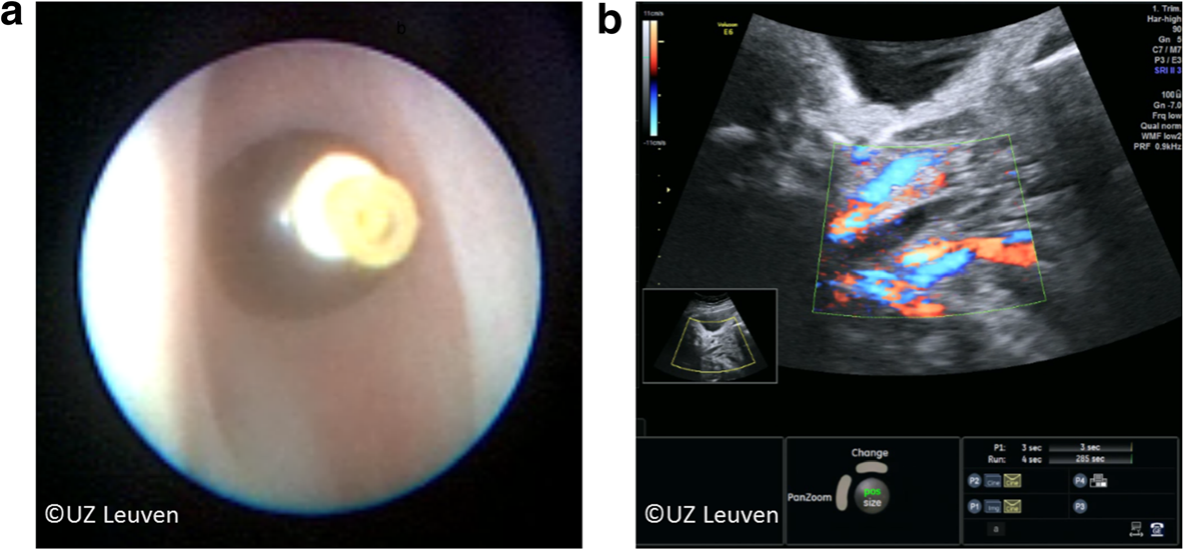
a After detachment the balloon can be seen through the vocal cords which are in this case just above it. b On ultrasound the balloon is visible as a hypoechogenic area. Power Doppler can help to distinguish the balloon from the adjacent blood vessels. *© UZ Leuven, UZ Leuven, Belgium*
